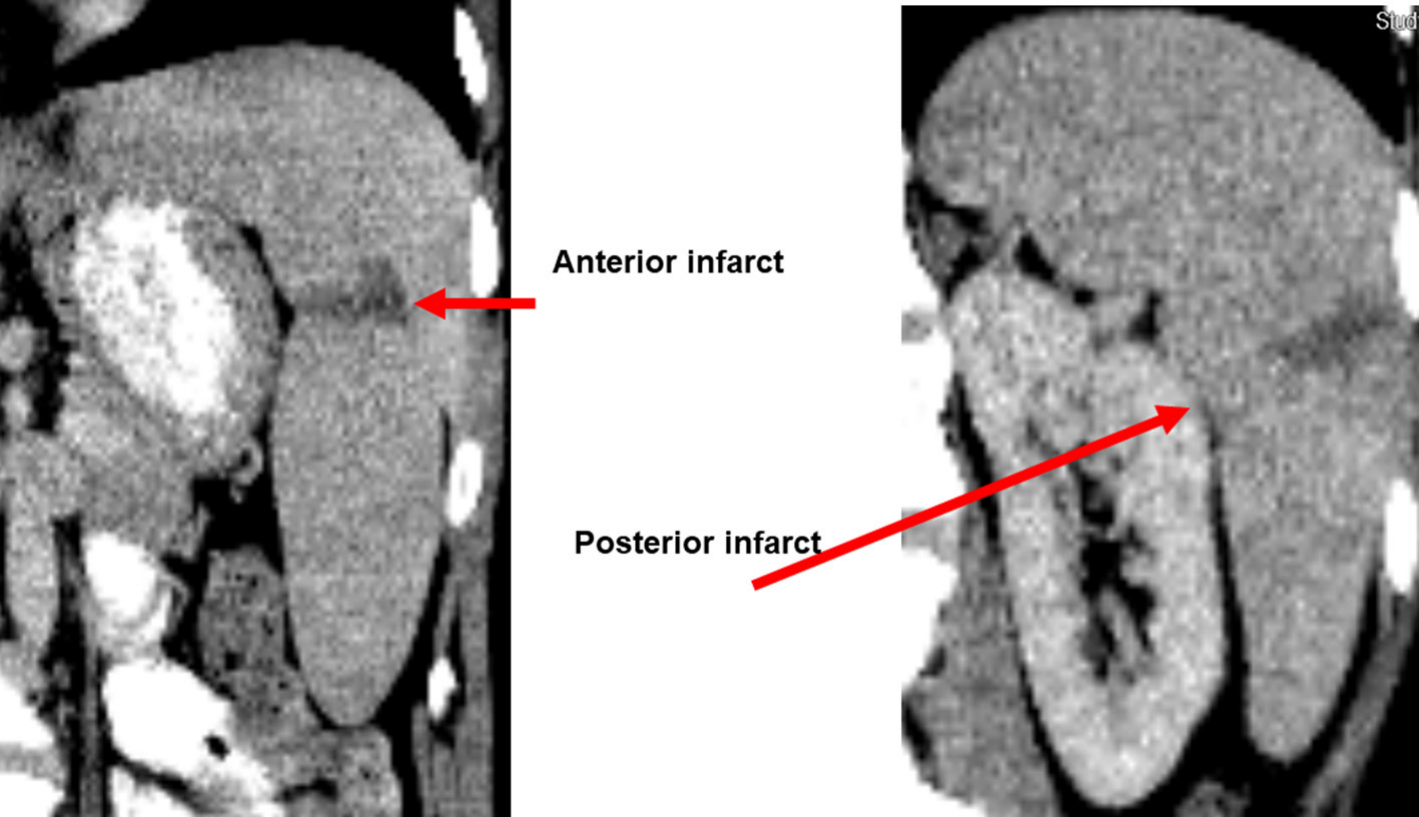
Contrast-enhanced CT scan of the abdomen showing evidence of splenic infarcts. (a) mild splenomegaly with wedge-shaped infarct in the anterior portion of the mid upper spleen; stable. (b) there is a similar wedge-shaped area of infarct in the posterior aspect of the spleen at the same level.
